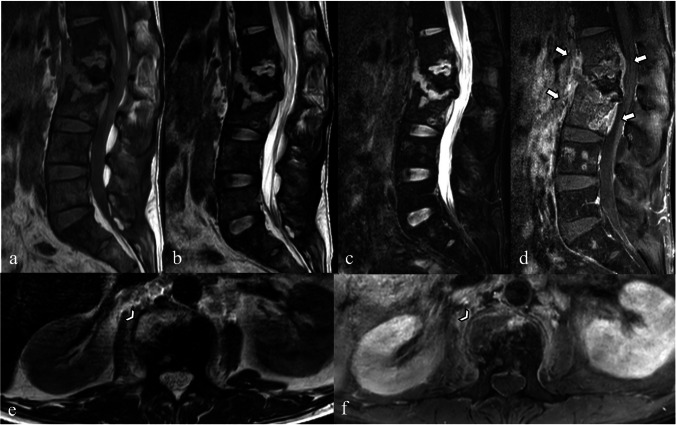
Magnetic resonance images of a 40-year-old male patient with melioidosis spondylodiscitis that mimics tuberculous infection. Sagittal T1-weighted (a), T2-weighted (b), short tau inversion recovery (c), and fat-suppressed contrast-enhanced T1-weighted (d) images show partially destroyed L1–L3 vertebrae, severely collapsed L2 vertebra with anterior subligamentous spread, and peri-vertebral and anterior epidural soft tissue involvements (arrows in d) causing moderate central spinal canal stenosis. Axial T2-weighted (e) and fat-suppressed contrast-enhanced T1-weighted (f) images demonstrate a small right paravertebral abscess (arrowheads)
